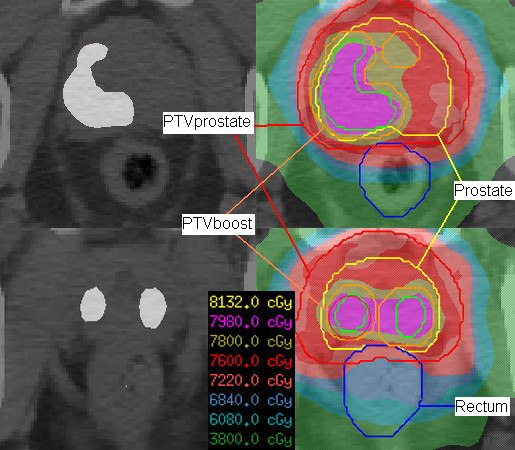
Simultaneous integrated boost concept for two patients with a PET-CT slice (images on the left, PET signal demonstrates the malignant lesion above a fixed standard uptake value threshold) and the corresponding contours and isodose lines in a treatment plan (images on the right).
